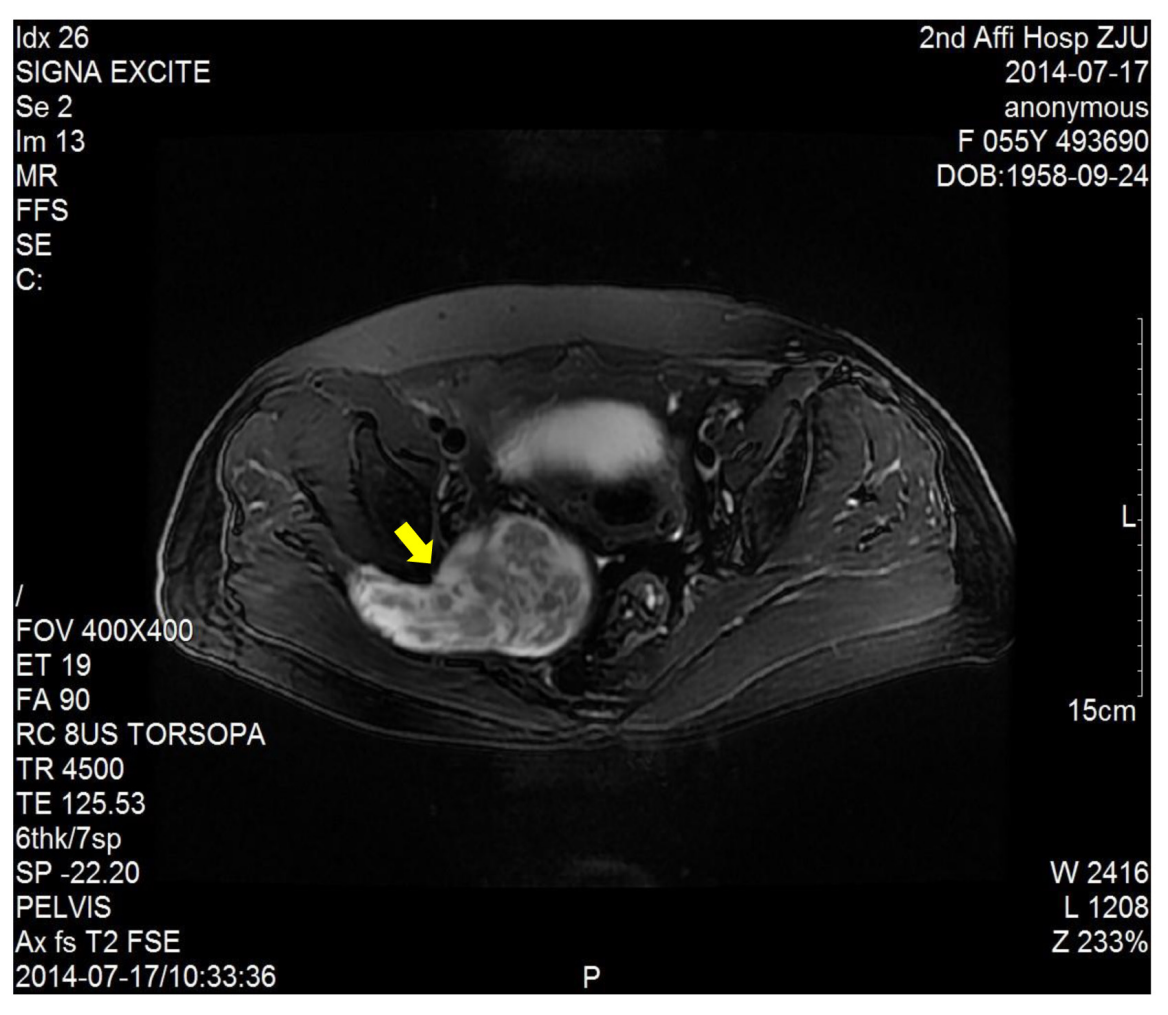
T2-weighted image showed a right sacral mass as a dumbbell-shaped configuration and mixed low signal intensity on the basis of high signal intensity
